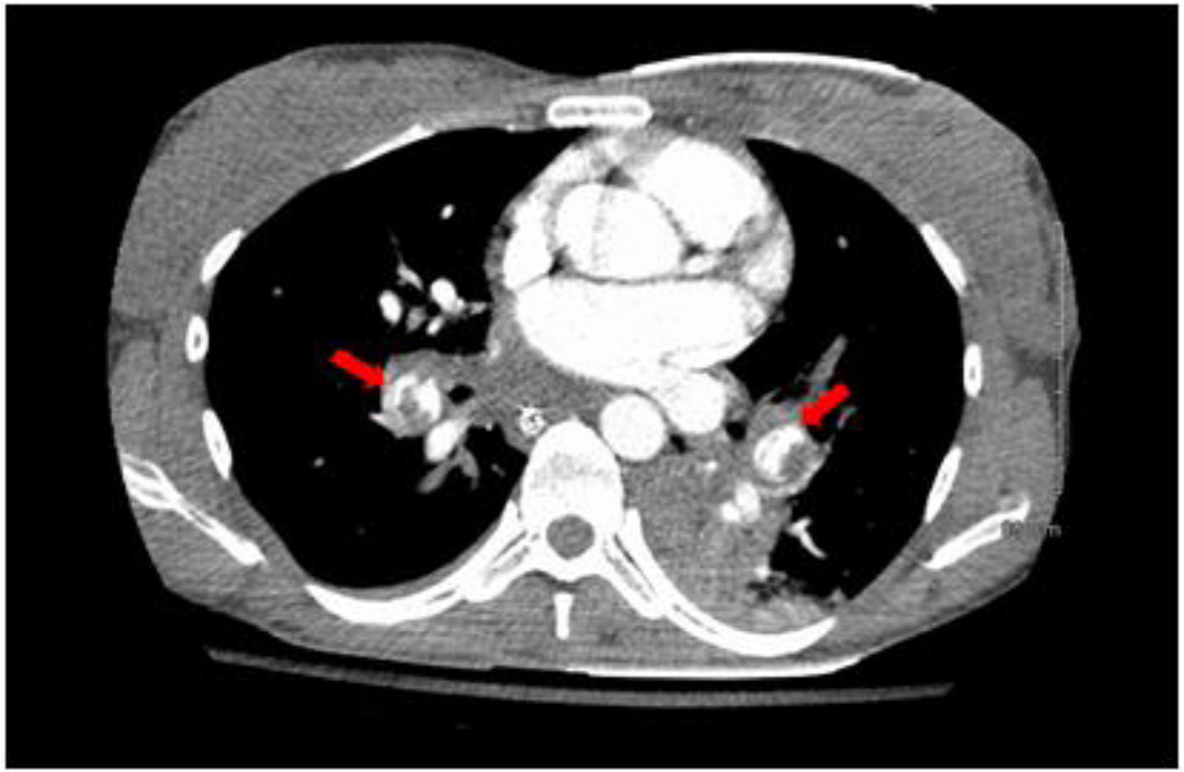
CT scan of the chest with contrast. The red arrows depict bilateral near occlusive pulmonary emboli
